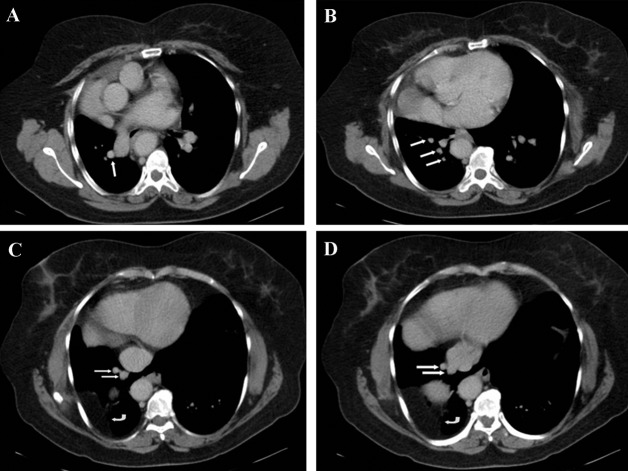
A, Contrast enhanced CT scan below the pulmonary sling in the same patient. The pulmonary vein is adjacent to the pulmonary artery (arrow). B, CT scan of the lung base. The branches of the anomalous venous structure are visible (arrows). C,D, Distal branches drained to the inferior vena cava (arrows). Abdominal fatty tissue and a part of the liver (curved arrow) are herniated to the posterobasal region of the thorax. (Bochdalek’s hernia)
